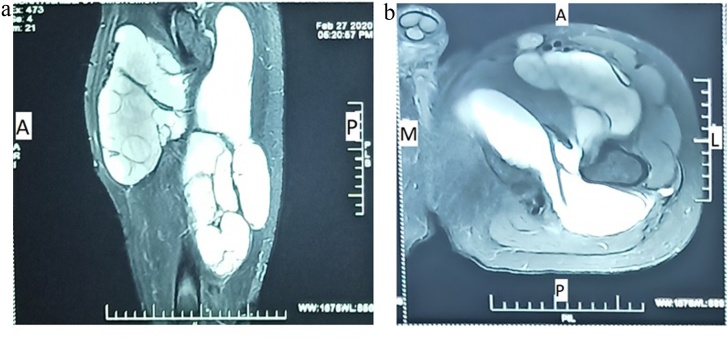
MRI showing intramuscular hydatid cyst with multiple daughter cysts in the left thigh (a) Coronal section (b) Axial section.
